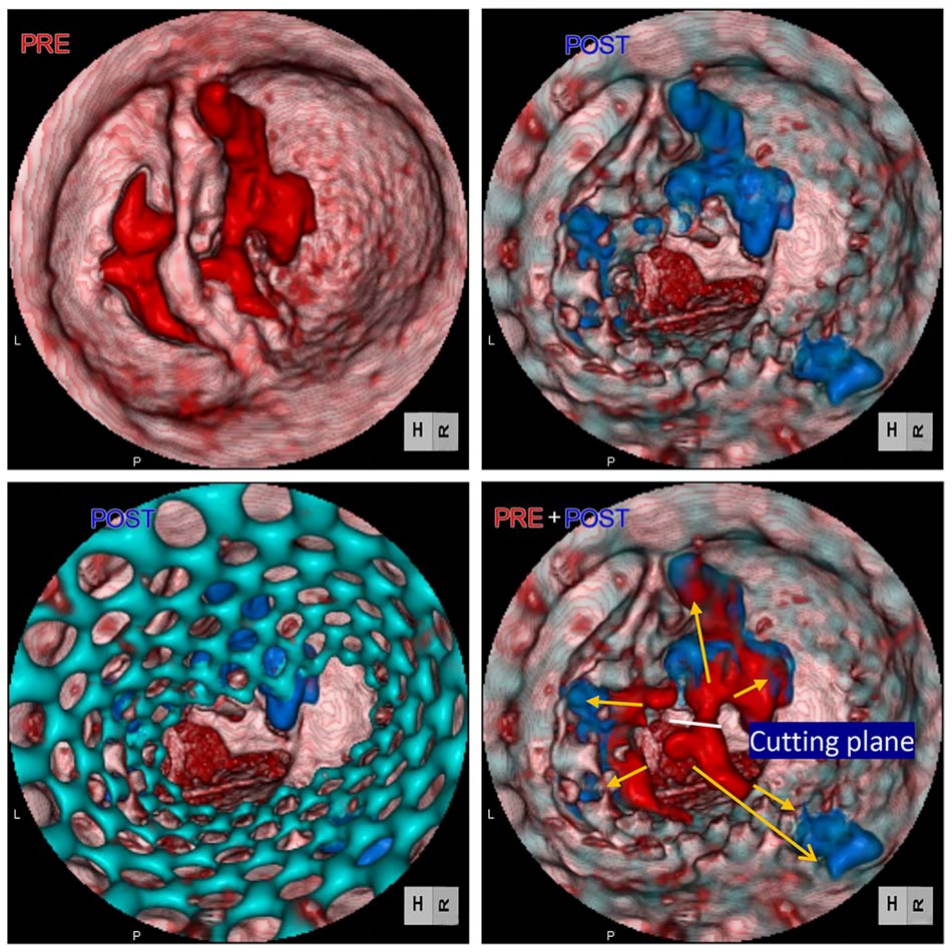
Computed tomography images of calcification before (red) and after (blue) TAVR. Distorted THV (green) at the annulus level was noted. Images were merged to detect the shifting calcification.
